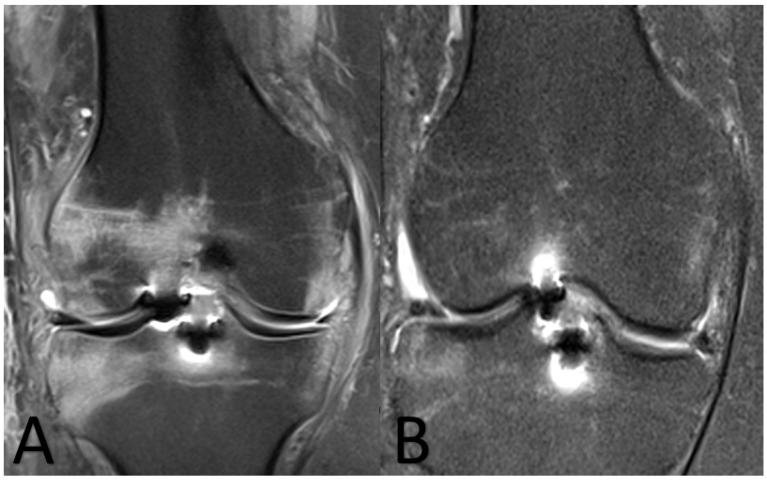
Coronal MRI views of the knee. (A) Fourteen days after the initial anterior cruciate ligament reconstruction; diffusely increased T2 signal/bone marrow edema is noted surrounding the ligament anchors, extending to the lateral femoral condyle, lateral tibial plateau, intercondylar eminences and the intercondylar notch. (B) Six months after antifungal treatment; imaging findings have notably subsided, indicating response to treatment.
